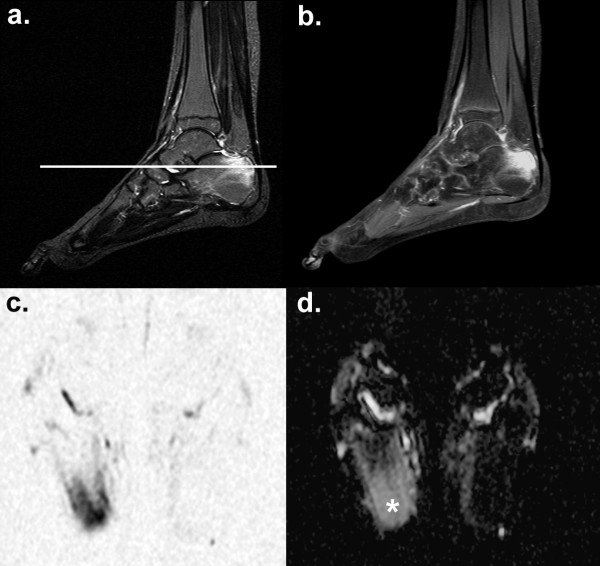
Bone marrow oedema in chronic non-bacterial osteomyelitis (CNO). A 12-year-old girl with multifocal CNO manifestations (rip bones, proximal femur, lower leg, metatarsal) shows intraosseous and paraosseal oedema on sagittal T2W TIRM (a), corresponding contrast enhancement on sagittal T1W TSE FS (b) and correlating signal changes on transversal DWI in the right calcanear tuber (c). The white line in (a) marks the cross-sectional plane of the DWI slice. Mean ADC of bone marrow oedema (white asterix) was 1.64 × 10^-3^ mm^2^/s (d).
